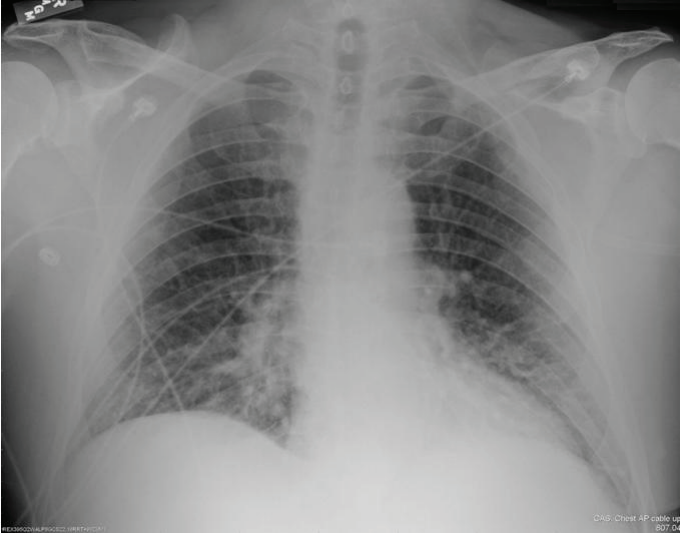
Chest radiograph at presentation
